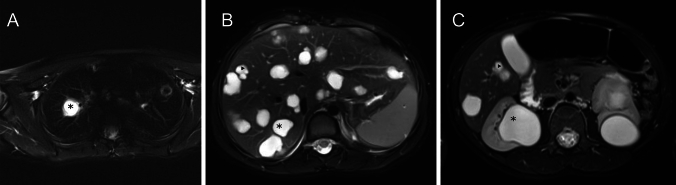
T2-weighted magnetic resonance imaging, performed prior to initiation of albendazole treatment showing multi-organ involvement with cysts in WHO stages CE1 (asterisks) and CE2 (arrowheads). a bipulmonary cysts b multiple hepatic cysts c bilateral kidney cysts
